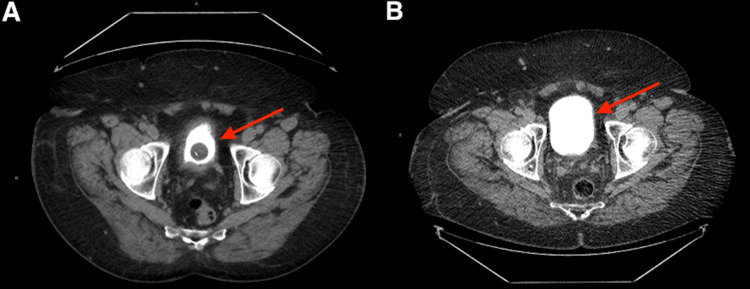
CT scans of the bladder taken one year apart, both without evidence of suspicious enhancement or masses. (A) Before induction of gemcitabine and pembrolizumab, with the arrow showing the bladder decompressed by a Foley catheter. (B) After discontinuation of pembrolizumab, with the arrow showing a partially distended bladder and no evidence of disease progression.
